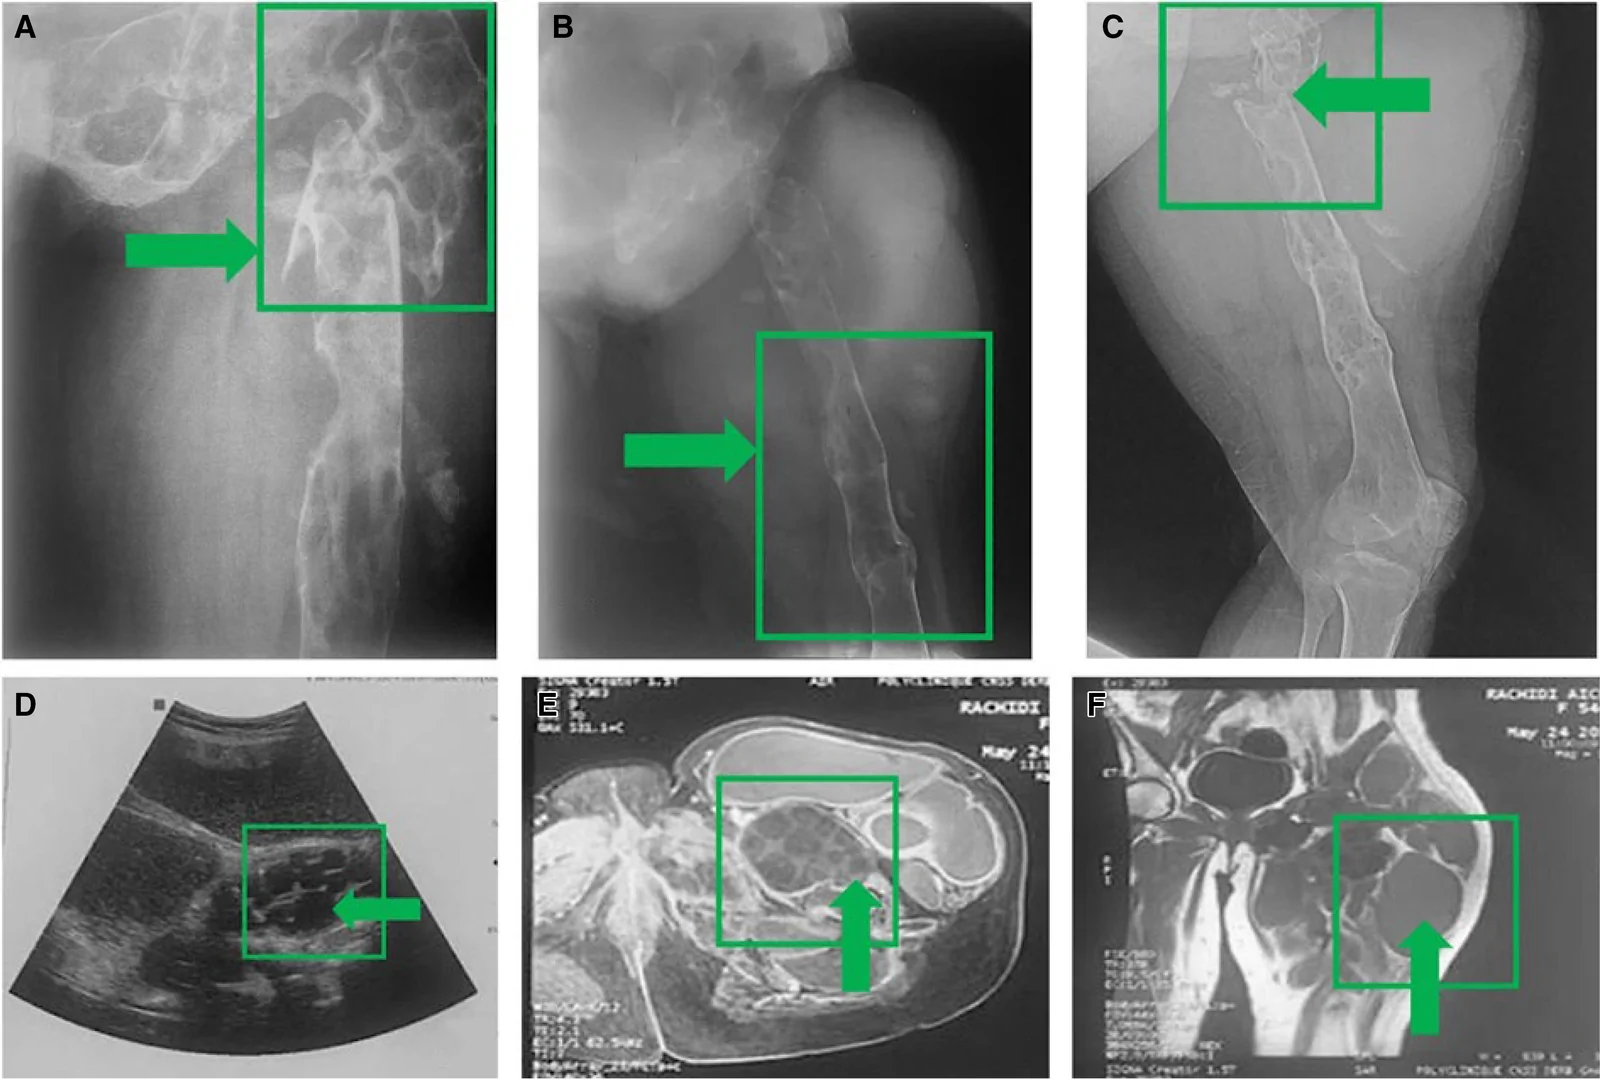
A cyst is shown in the X-rays and magnetic resonance images (MRIs) of the upper-left thigh and hip area. (A–C) Lytic lacunar lesions clustered to form a honeycomb pattern, with thinning and a break in the cortical bone, as well as a pathological fracture located in the subtrochanteric region. (D) A cyst shown in an ultrasound image displaying. (E and F) An MRI of the thigh revealed a multilocular lesion measuring 26.5 cm by 18.7 cm, along with a cystic mass at the root of the thigh.
